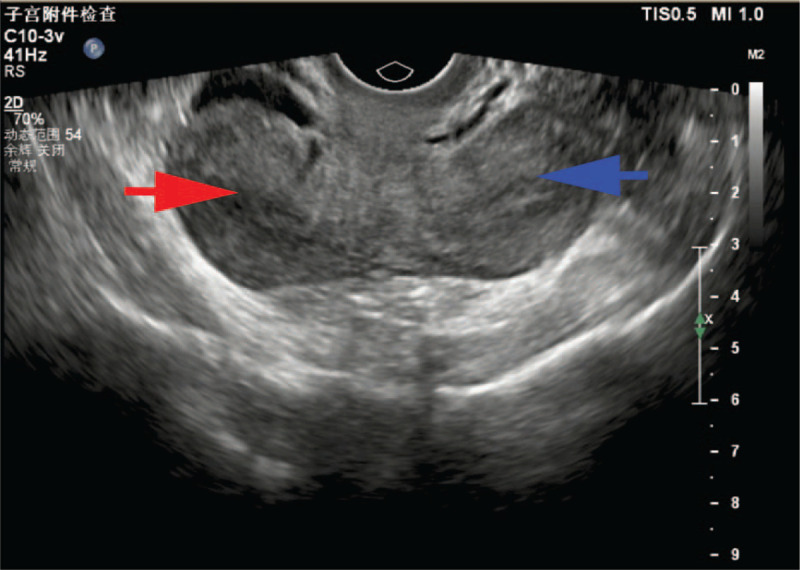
Transvaginal ultrasound postsurgery confirmed a didelphic uterus with no mass in the bilateral adnexa regions. The red arrow indicates the right uterus. The blue arrow indicates the left uterus.
